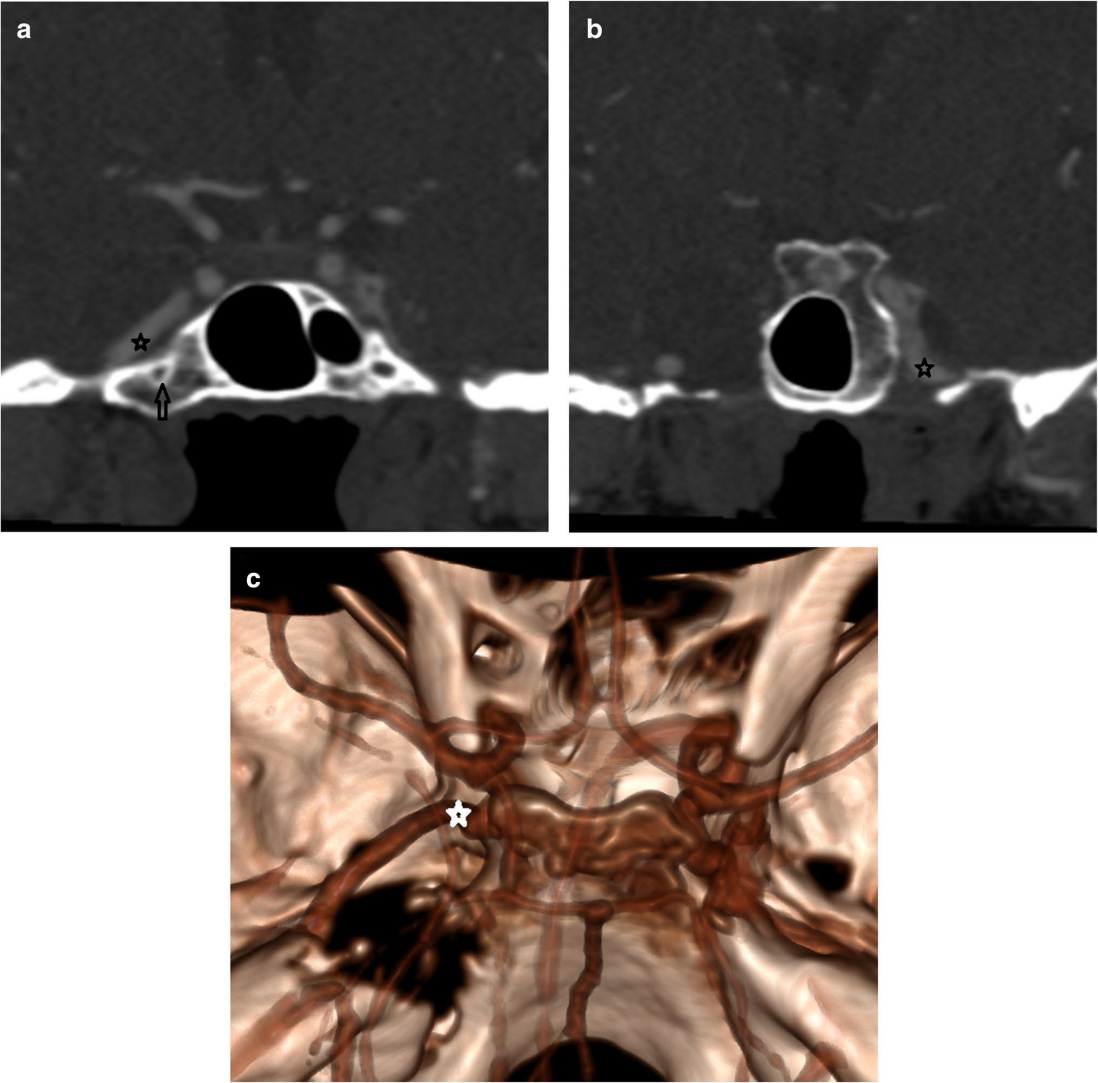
Patient 6. (a) Coronal reformat of the CTA perpendicular to the craniocaudal angle of the VC shows the displaced right anterior genu of the pICA (black star) to lie superolateral to the VC and demonstrating a shallow curve (black open arrow) on the chondrosarcoma side. (b) More posterior coronal reformat of the CTA shows the left anterior genu of the pICA (black star) to lie superomedial to the VC on the normal side with a better defined angle. (c) Volume-rendered image was used to locate the apex of the anterior genu (white star) when distorted
